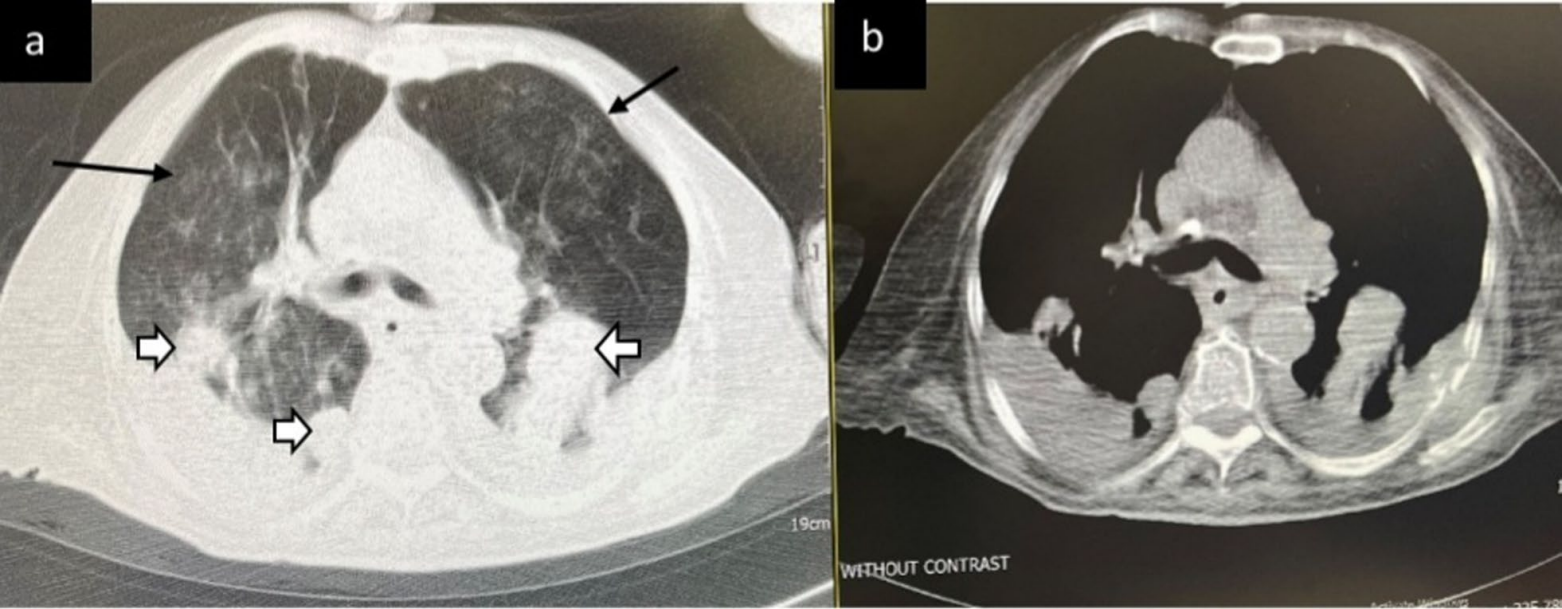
Indeterminate CT imaging features for COVID-19. Unenhanced, axial images of the lung and mediastinal window (a, b) in an 83-year-old woman with colon cancer and positive RT-PCR shows few, small GGO with a non-rounded and non-peripheral distribution (black arrows) with metastatic nodules and mass (white arrows) and pleural effusion (b)
